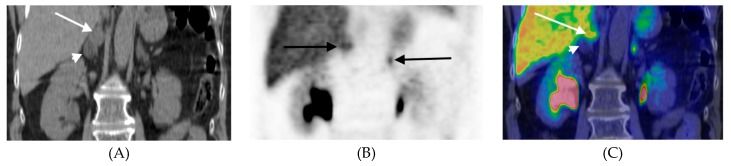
^11^C-HED-PET/CT, coronal mages, of patient #2 (Table 3) with a collision tumor in the right adrenal showing a cranial component with high tracer uptake comprising a PCC (arrows) and a caudal tumor portion representing an adrenocortical adenoma (arrow head). The normal contralateral adrenal is indicated with an arrow in the PET image. (A) CT, (B) PET, (C) PET/CT fusion.
